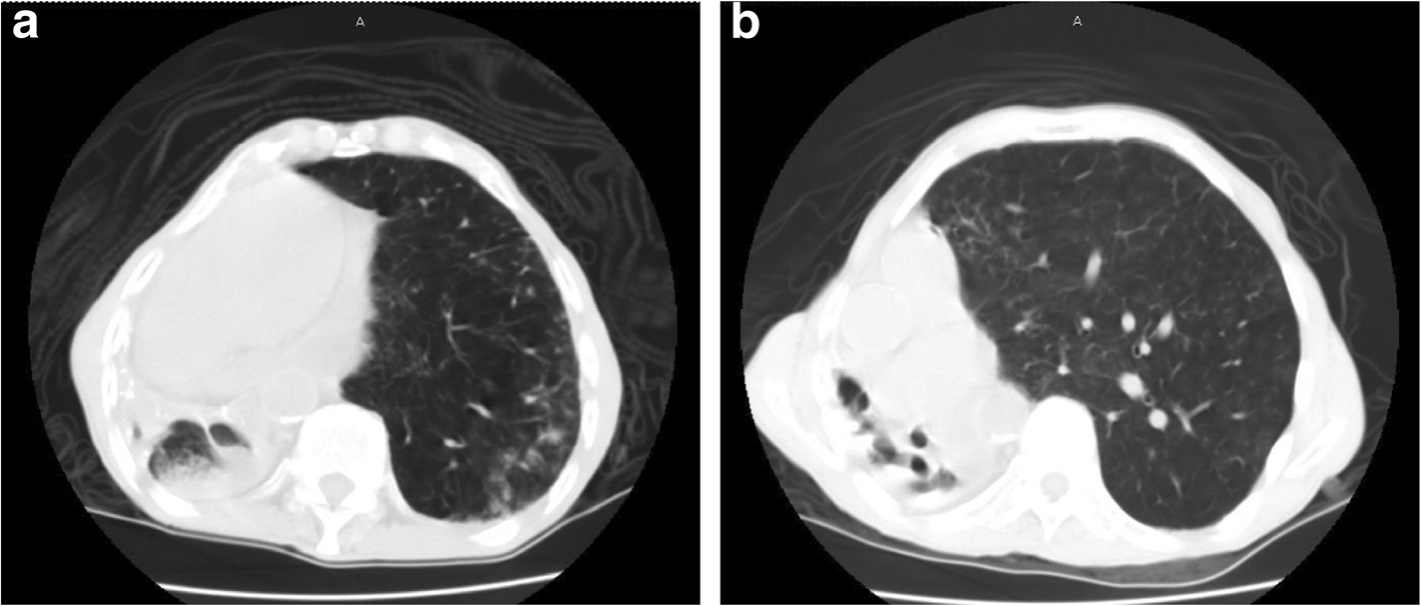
(a) Computed tomography scan of the chest showing counterclockwise rotation of the mediastinum and great vessels. (b) Computed tomography scan of the chest showing counterclockwise rotation of the mediastinum and great vessels; the trachea is to the right of the spine.
